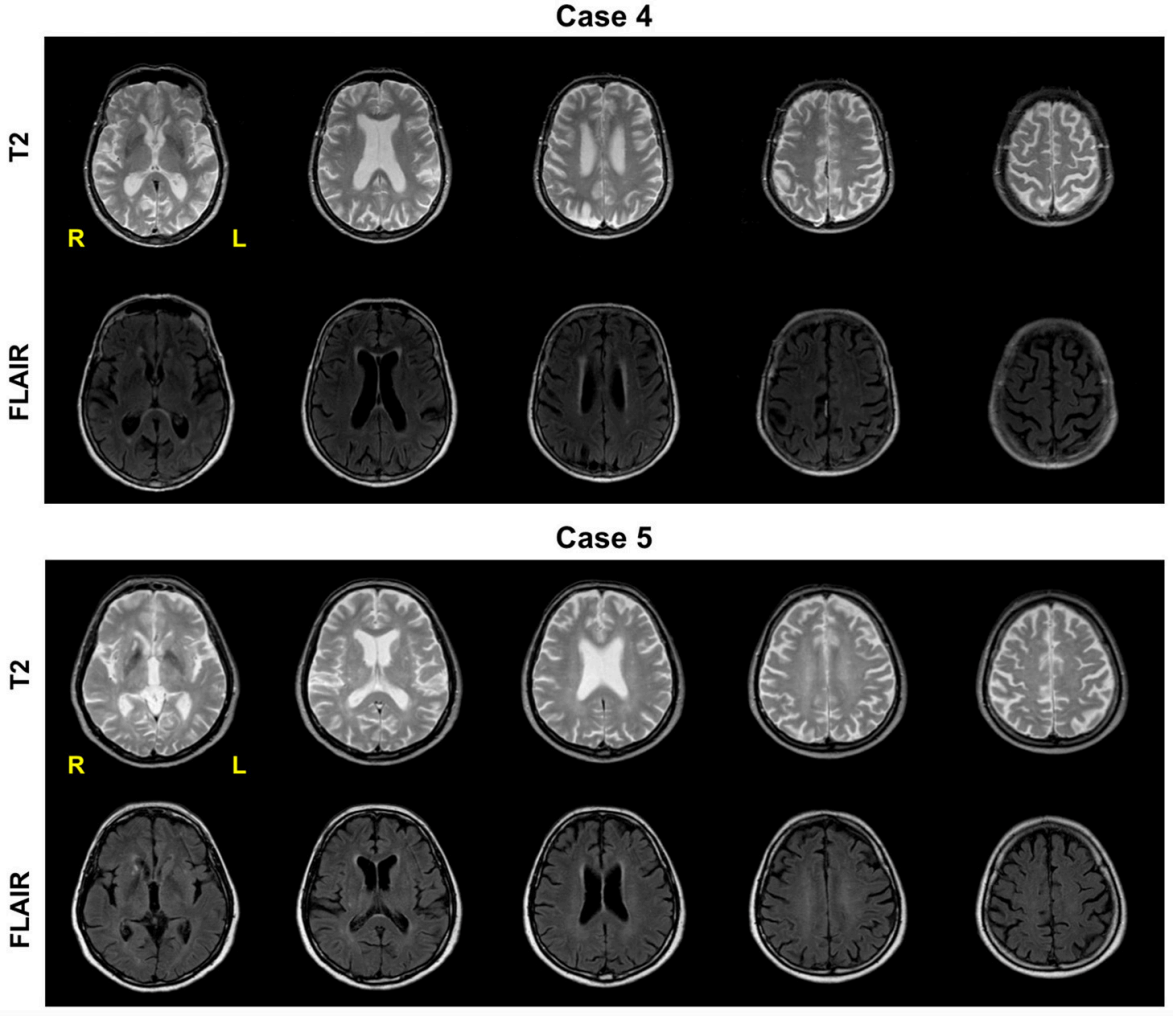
Axial T2-weighted (top) and FLAIR (bottom) MR images for Case 4 and Case 5.
